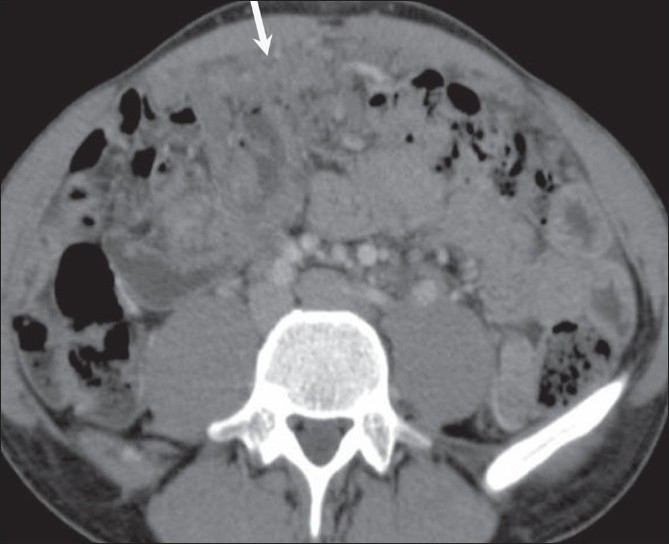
Tuberculosis. Axial, contrast-enhanced CT scan in a 35-year-old man with abdominal tuberculosis shows diffuse omental thickening (arrow)
